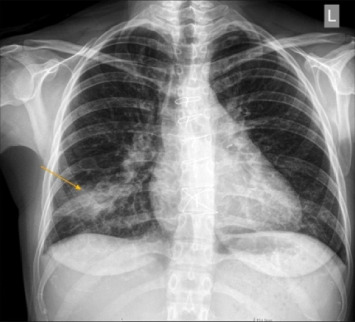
Chest x-ray revealed consolidation of right lower lobe (yellow arrow).
